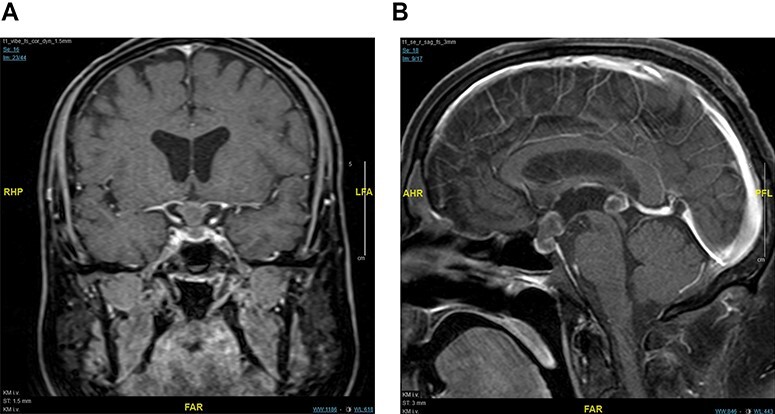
Image A, coronal MRI with intravenous contrast depicting a pituitary stalk metastasis (white arrow). Image B, sagittal MRI with intravenous contrast depicting metastases to the pituitary stalk (white arrow) and pineal gland (white arrowhead).
